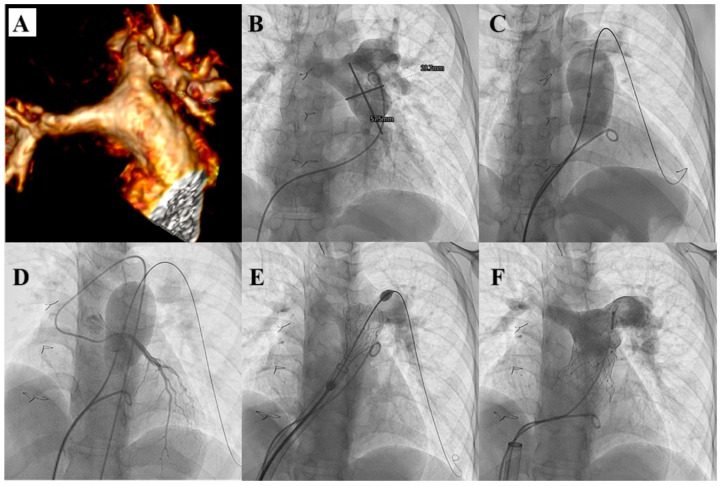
Venus P-Valve implantation. Three-dimensional angio CT scan of a large native RVOT in a repaired tetralogy of Fallot with significant pulmonary regurgitation (A). Pulmonary angiography detects the effective diameter and length of the native RVOT (B). RVOT balloon interrogation with a compliant balloon demonstrates a 32 mm waist as a landing zone (C). RVOT balloon interrogation with a semi-compliant balloon excludes coronary artery compression (D). Venus P-Valve implantation (E) and pulmonary angiography demonstrating a continent pulmonary valve (F).
